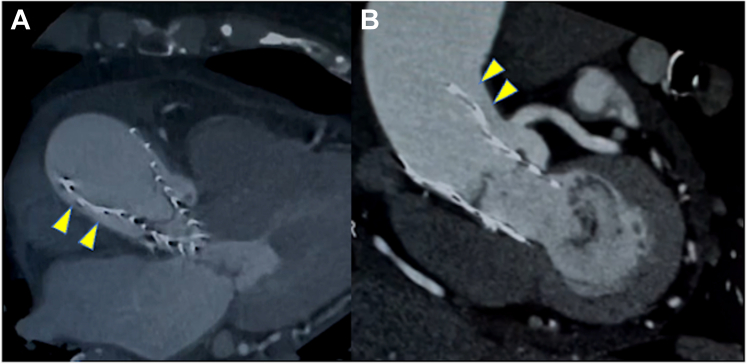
CT of Aortic Root and Ascending Aorta (A) Axial view and (B) coronal view CT revealing localized dissection of the ascending aorta at the superior border of the Evolut PRO+ stent (arrowheads). CT = computed tomography.
